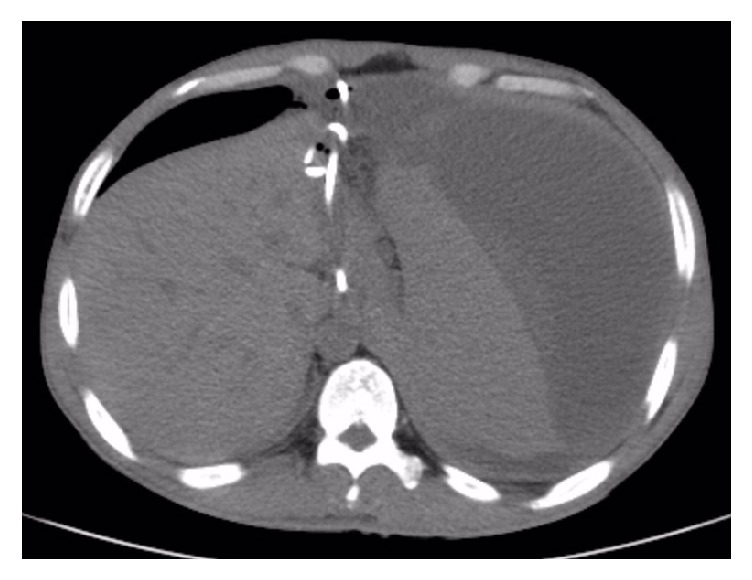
CT showing the drainage tube was in place, but splenic subcapsular hematoma had no change.
